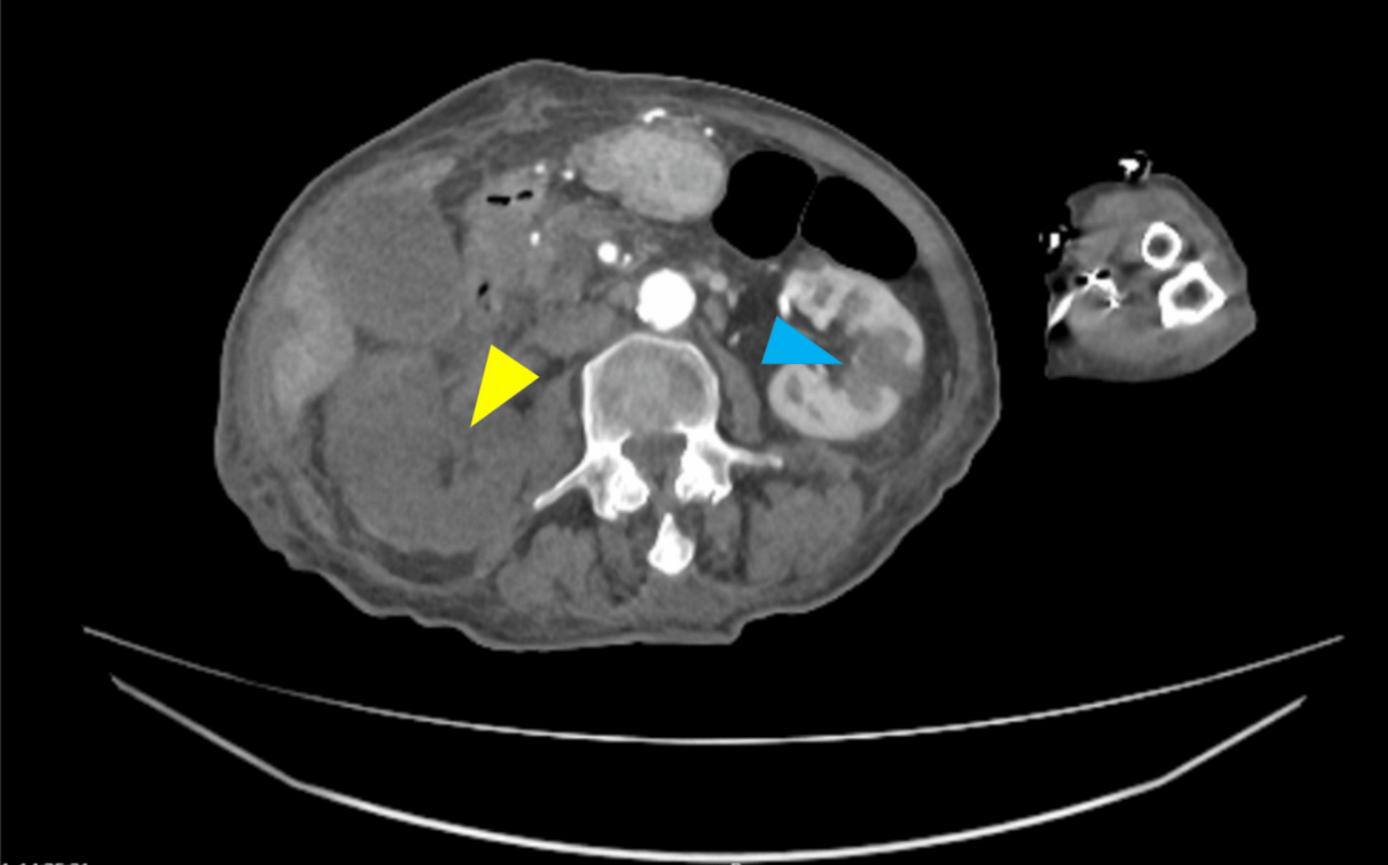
CT angiogram showing a complete lack of enhancement of the right kidney (yellow arrow) and segmental occlusion of the left kidney (blue arrow).
